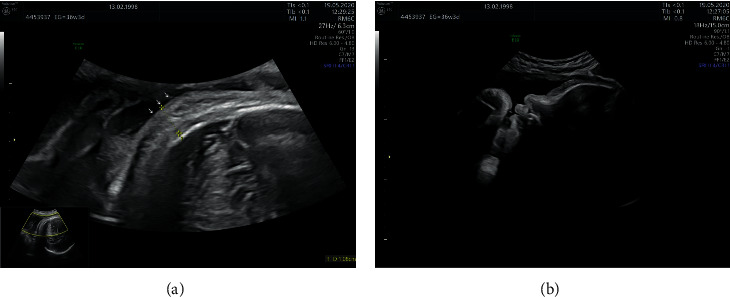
(a) A transitory fetal skin edema (11 mm) was visualized in the axial plane of the fetal head by ultrasound at 36 3/7 weeks of pregnancy. (b) Sagittal view of the transitory fetal head skin edema at 36 3/7 weeks of pregnancy.
